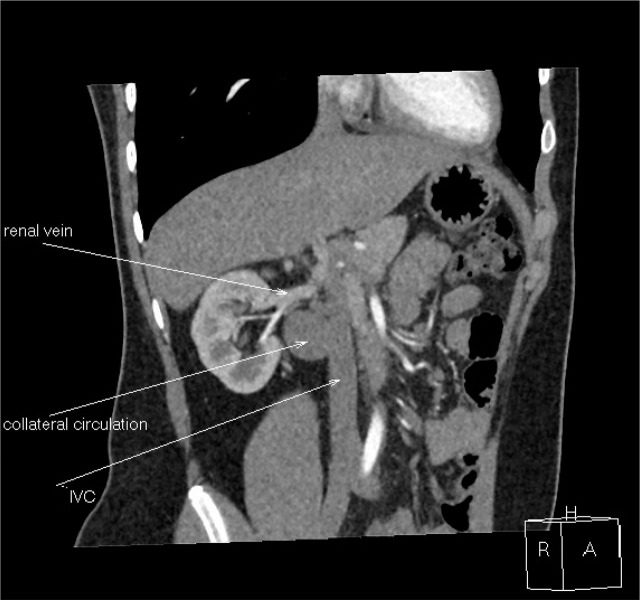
CT scan of abdomen: Lack of hepatic and suprarenal IVC, venous outflow leads through vena azygos and hemiazygos.
